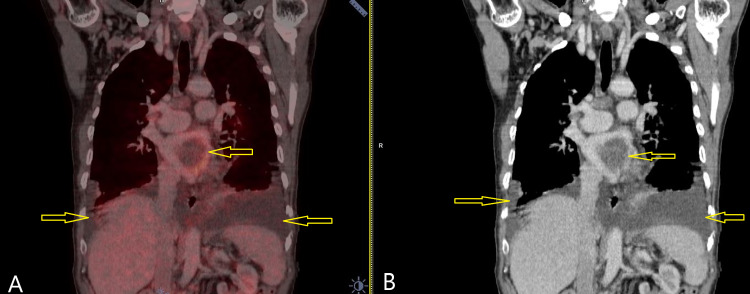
Nodules in bilateral lung fields with bilateral moderate pleural effusion and thrombus in left atrium extending into pulmonary veins. Seen with Fluorodeoxyglucose (FDG) avidity in fused PET-CT (A) as well as in contrast CT (B).
